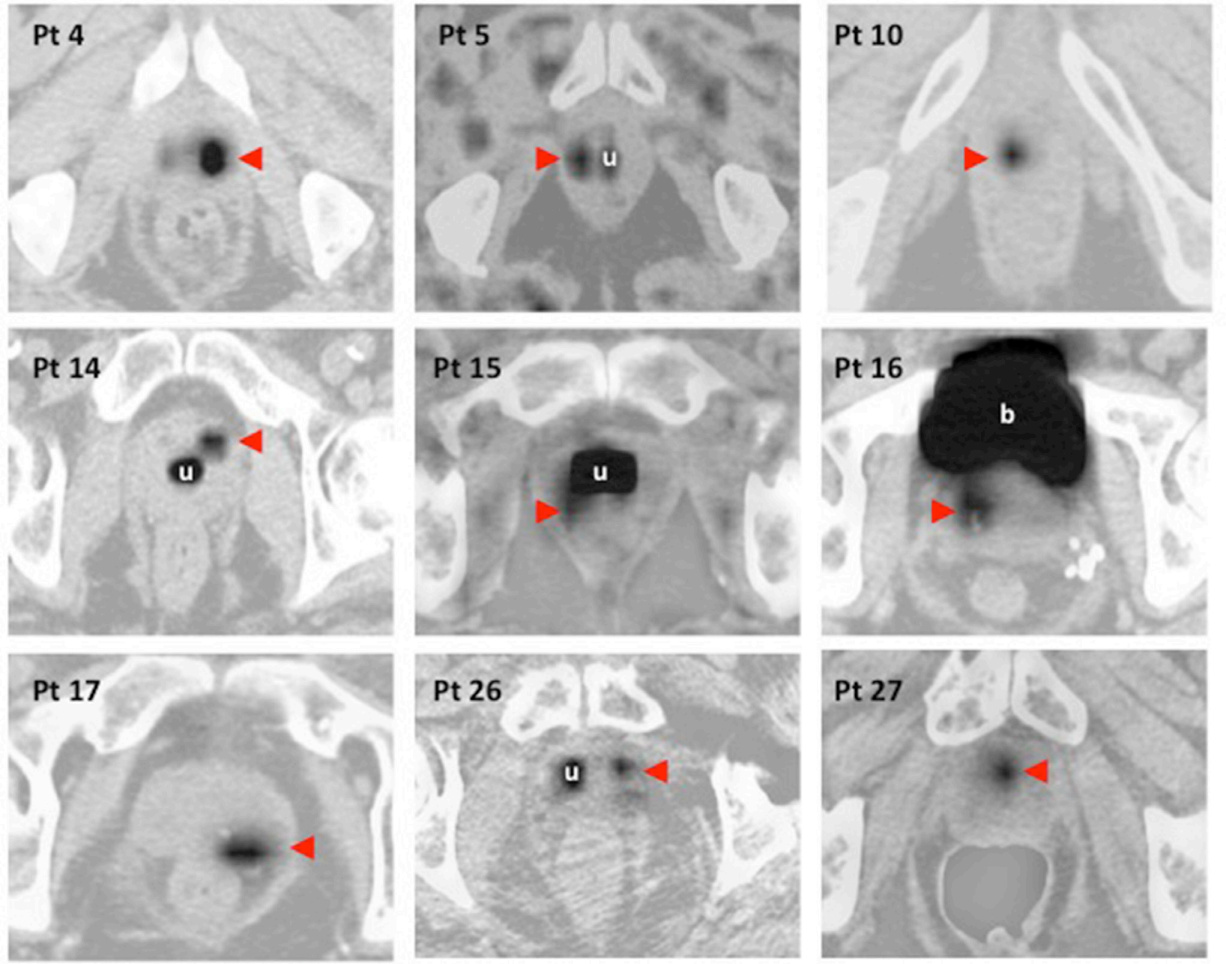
Native FMISO uptake images and attenuation-corrected FMISO images fused with CT-scan images Eleven FMISO-positive regions (red arrows) were determined in 9 out of 27 patients. u: urethra; b: bladder. In patient 5 and 26, only one FMISO-positive region is shown.
